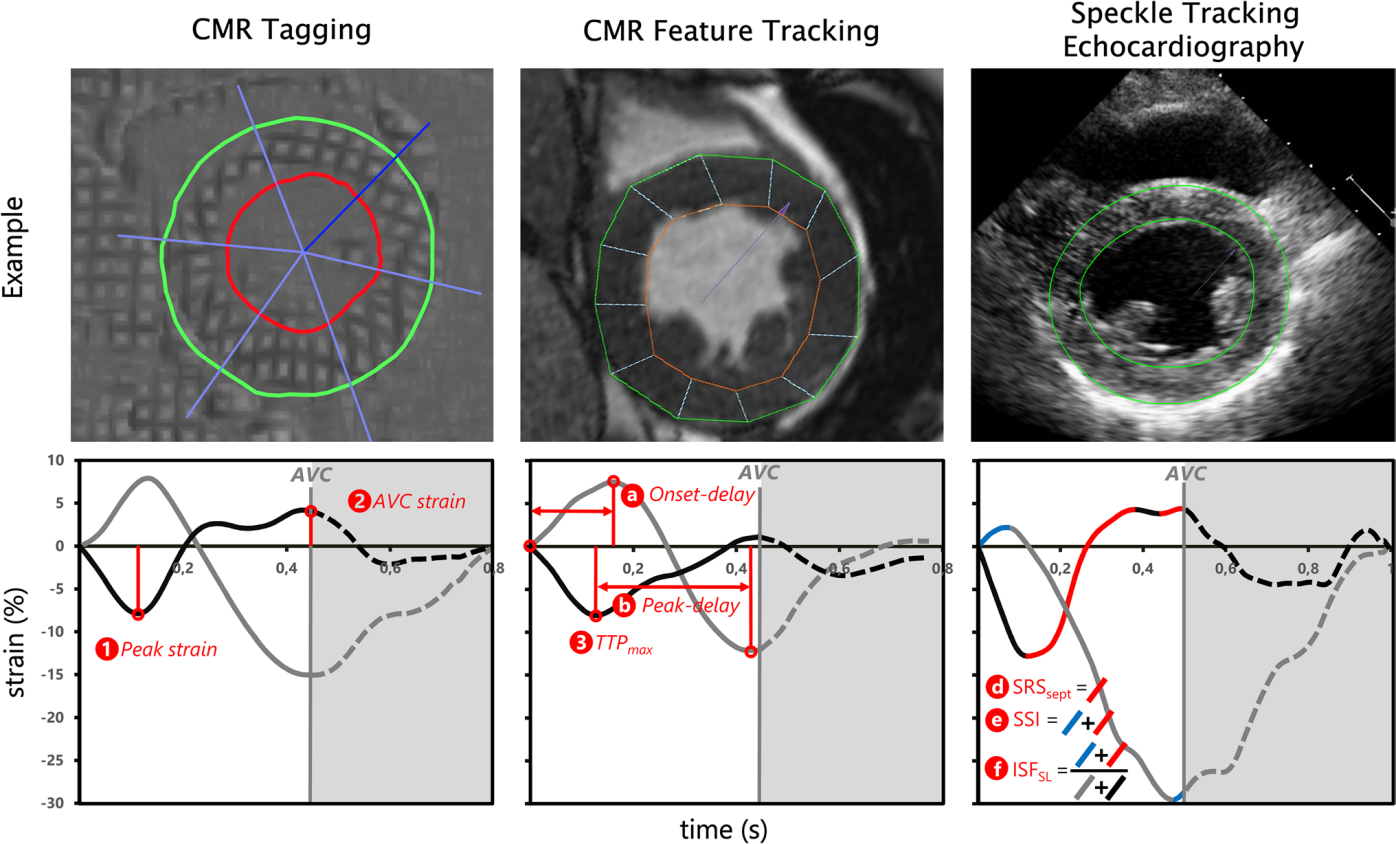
Overview of imaging techniques and corresponding myocardial strain analysis. Examples of imaging techniques (*top* row) and resulting strain signals (*bottom* row) of one specific patient. Each column represents a single technique with the corresponding strain results. Examples of derived parameters are shown per graph. Basic strain parameters are indicated with a number, dyssynchrony and discoordination parameters are indicated with a character. Strain signals of the septum (black line) and lateral wall (grey line) are given, with the aortic valve closure (grey vertical line) as end of systole. *CMR* cardiac magnetic resonance imaging, *AVC* aortic valve closure, *AVC strain* strain value at aortic valve closure, *TTP*
